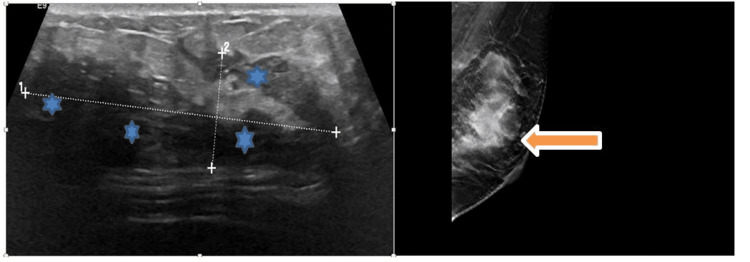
Left: Irregular left breast mass measuring 5.5 x 1.7 cm observed on ultrasound (indicated by stars). Right: mammography showing the same lesion (orange arrow).
